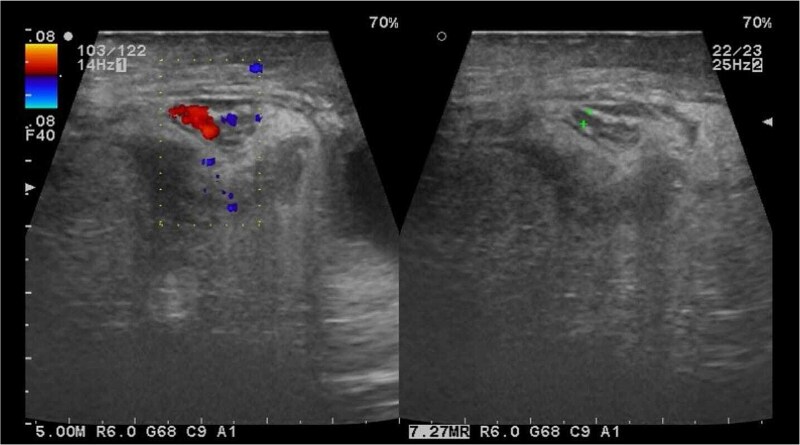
Testicular ultrasound with intestinal loops.
